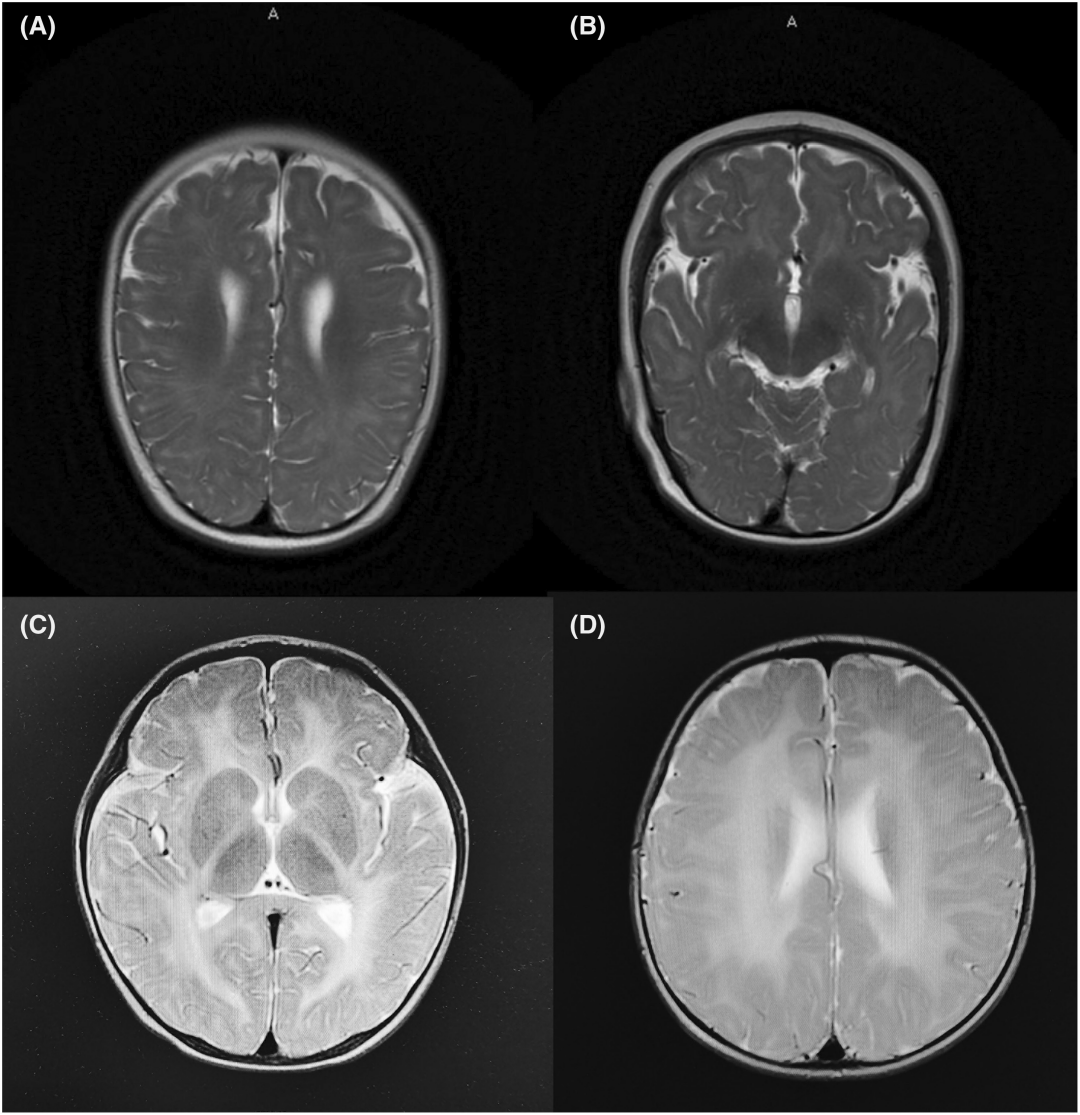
Brain MRI findings in patients with RARS1 biallelic variants. (A,B) Patient 1's axial T2‐weighted imaging showed an abnormal T2 hyperintense signal of the cerebral white matter and simplified gyral pattern. (C,D) Patient 2's axial T2‐weighted imaging showed an abnormal T2 hyperintense signal of cerebral white matter.
